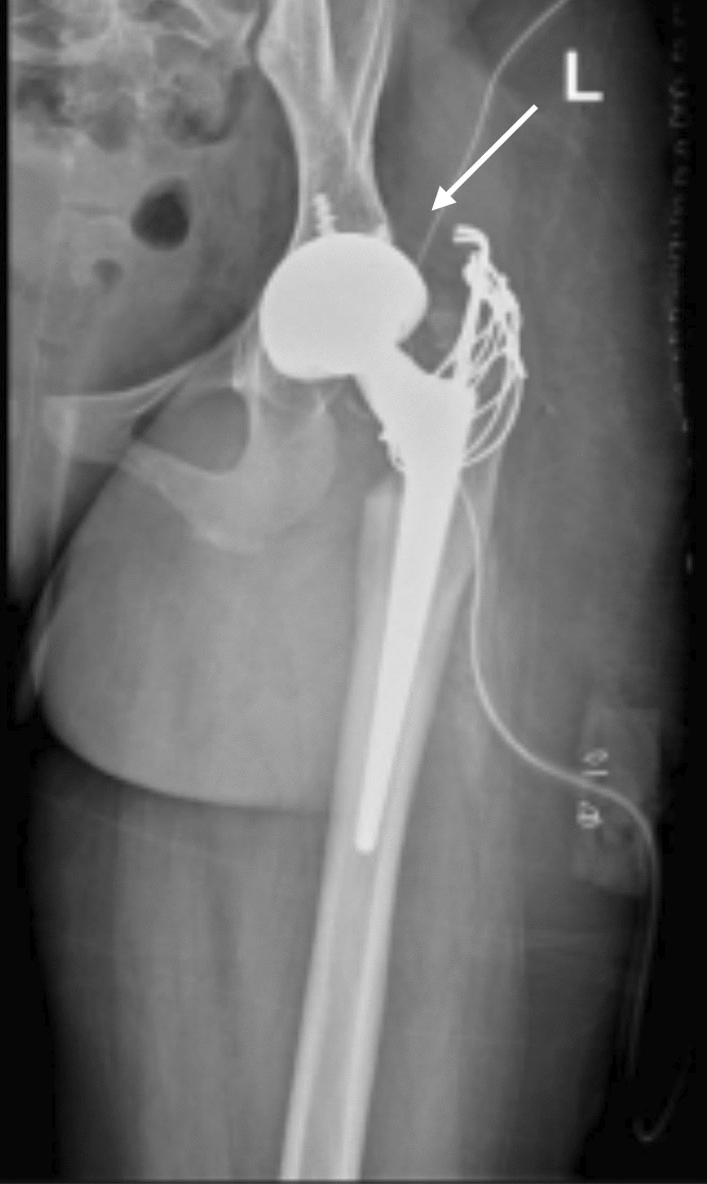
X-ray of left hip showed the implant well positioned and with good alignment and there is no obvious osteolysis or dislocation or any pressure was seen on the left hip
